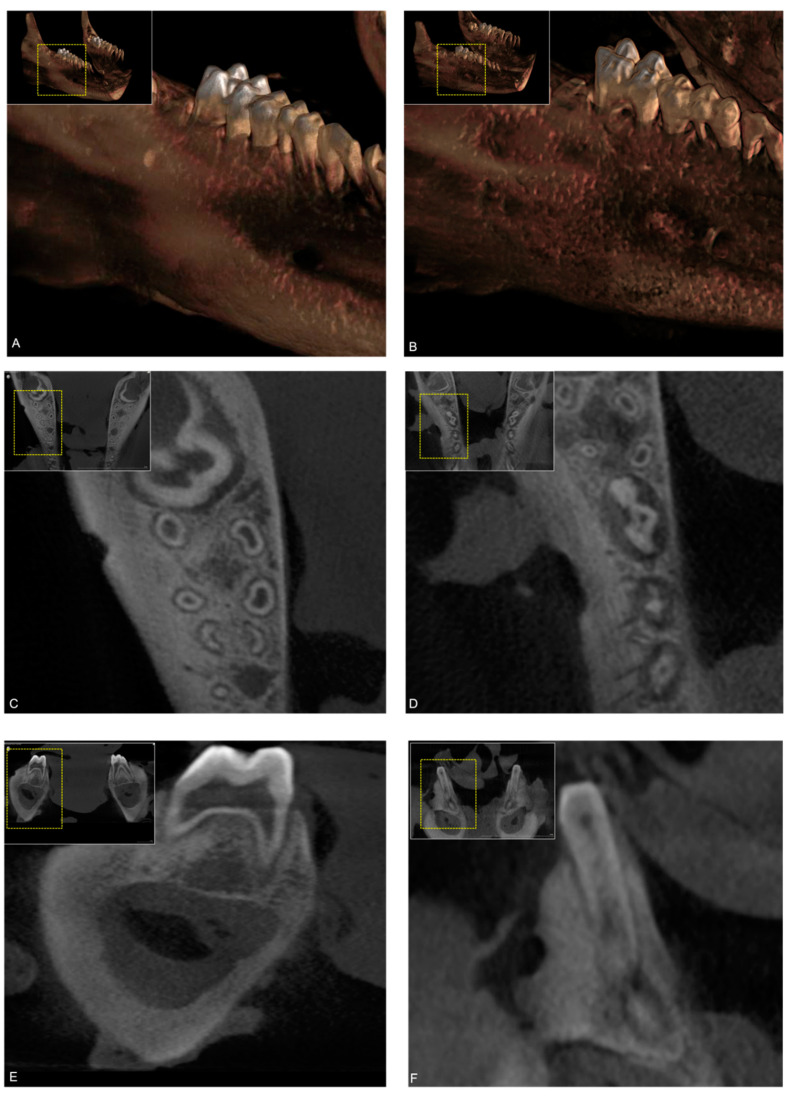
Screenshots of the cystic lesions visualized using the standard-dose (upper row) (image reconstruction (A), axial (C), and coronal (E)) and low-dose (lower row) (image reconstruction (B), axial (D), and coronal (F)) cone-beam computed tomography (CBCT) imaging protocols.
